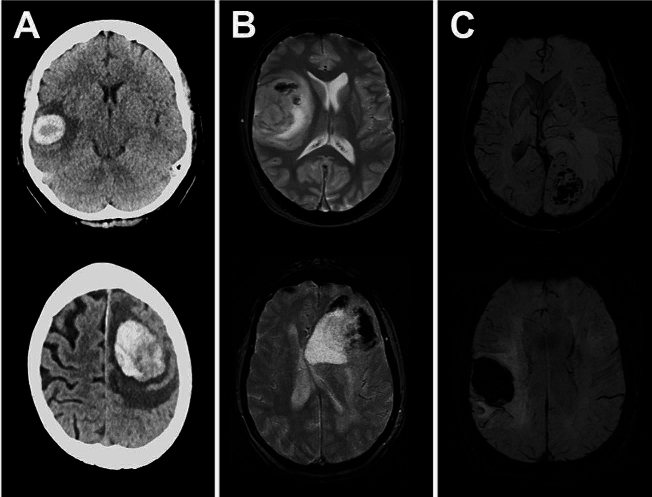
Exemplary cases of patients with hemorrhagic BM. Shown are 6 different patients with hemorrhagic brain metastases, using (A) CT imaging, (B) MRI / GRE-T2* imaging, and (C) MRI / SWI imaging. The axial slices are shown
